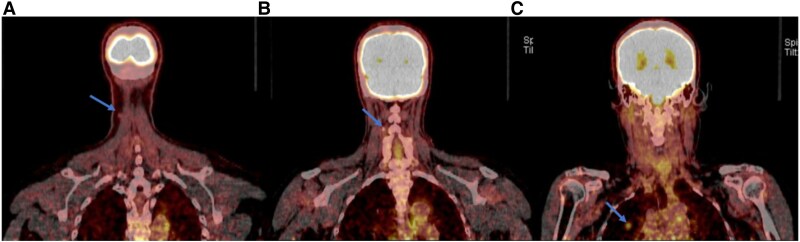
Positron emission tomography/computed tomography imaging from the skull to mid-thigh reveals hypermetabolic activity in the cutaneous lesion in the right anterior lower neck (arrow) (A), the lymph node lesion in the right anterior lower neck (arrow) (B), and the nodule in the right upper lobe of the lung (arrow) (C).
